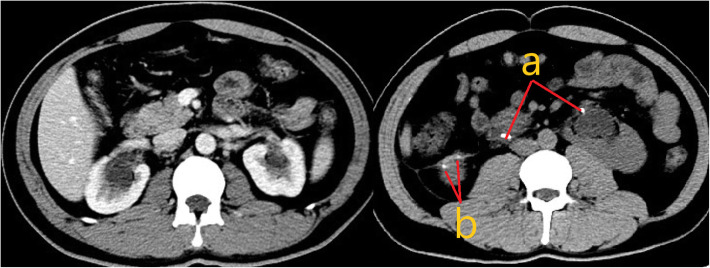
Postoperative computed tomography shows a few inflammatory changes and hydronephrosis for both kidneys: a) bilateral ureteral stent. b) postoperative changes after use of a laparoscopic linear incision stapler
